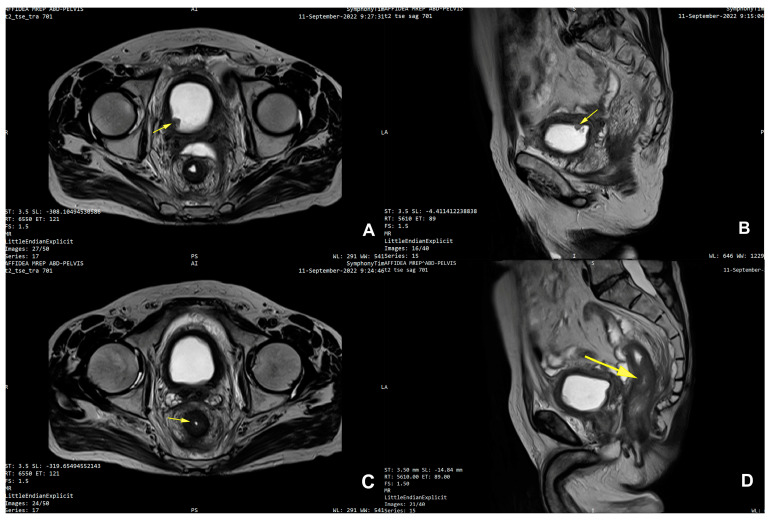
(A) Axial MRI diagnostic images with diffuse thickening of urinary bladder wall, non-contiguous with rectal tumor and arrow pointingthe small bladder tumor on the right postero-lateral wall; (B) sagittal MRI diagnostic images with arrow pointingthe bladder tumor; (C) axial MRI diagnostic images with arrow pointing the circumferential rectal thickening and concentric luminal stenosis; (D) sagittal MRI diagnostic images with arrow pointing the rectal tumoral stenosis.
